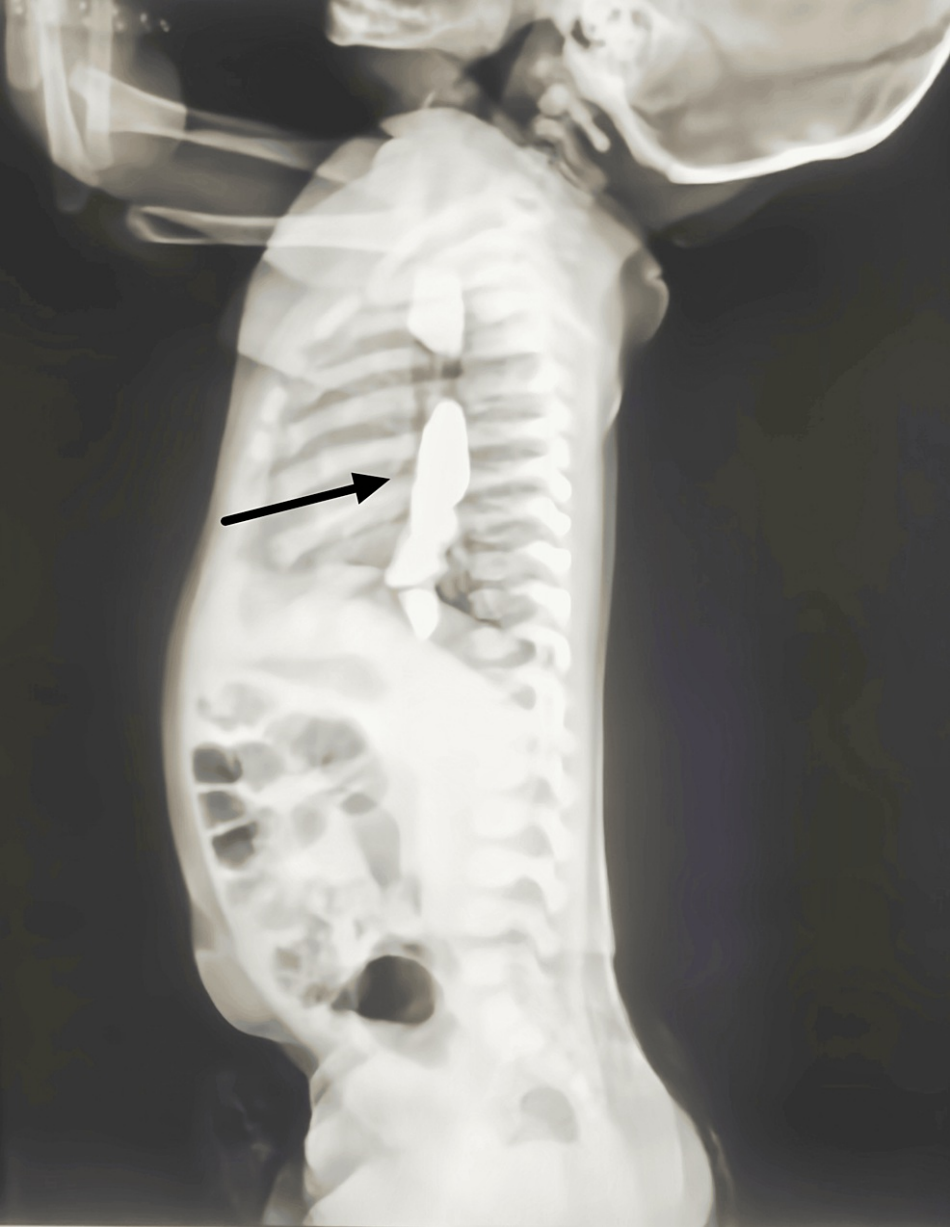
CT thorax image of the esophageal foreign bodies
